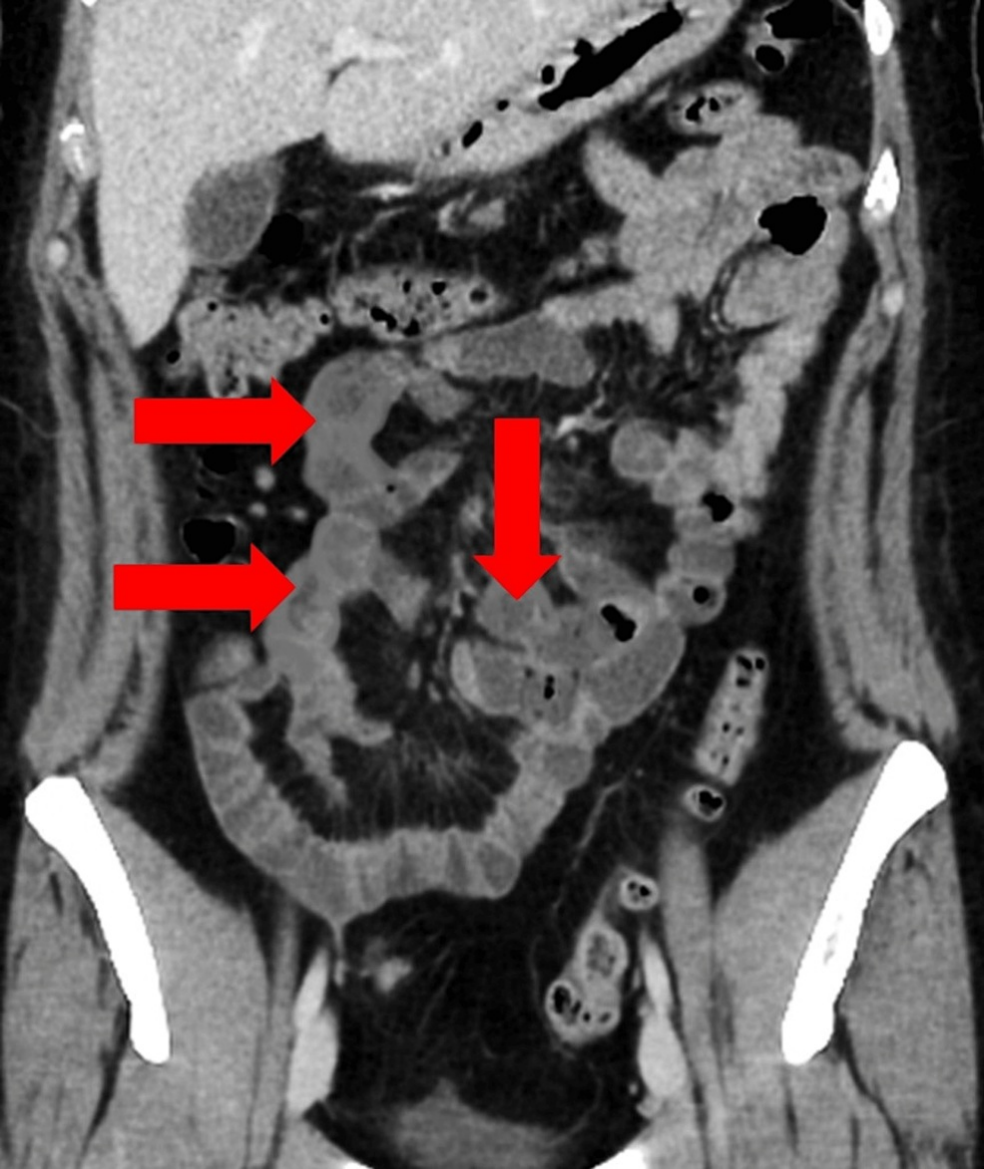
CT scan of the abdomen showing segmental thickening of the duodenum and jejunum gut walls.
